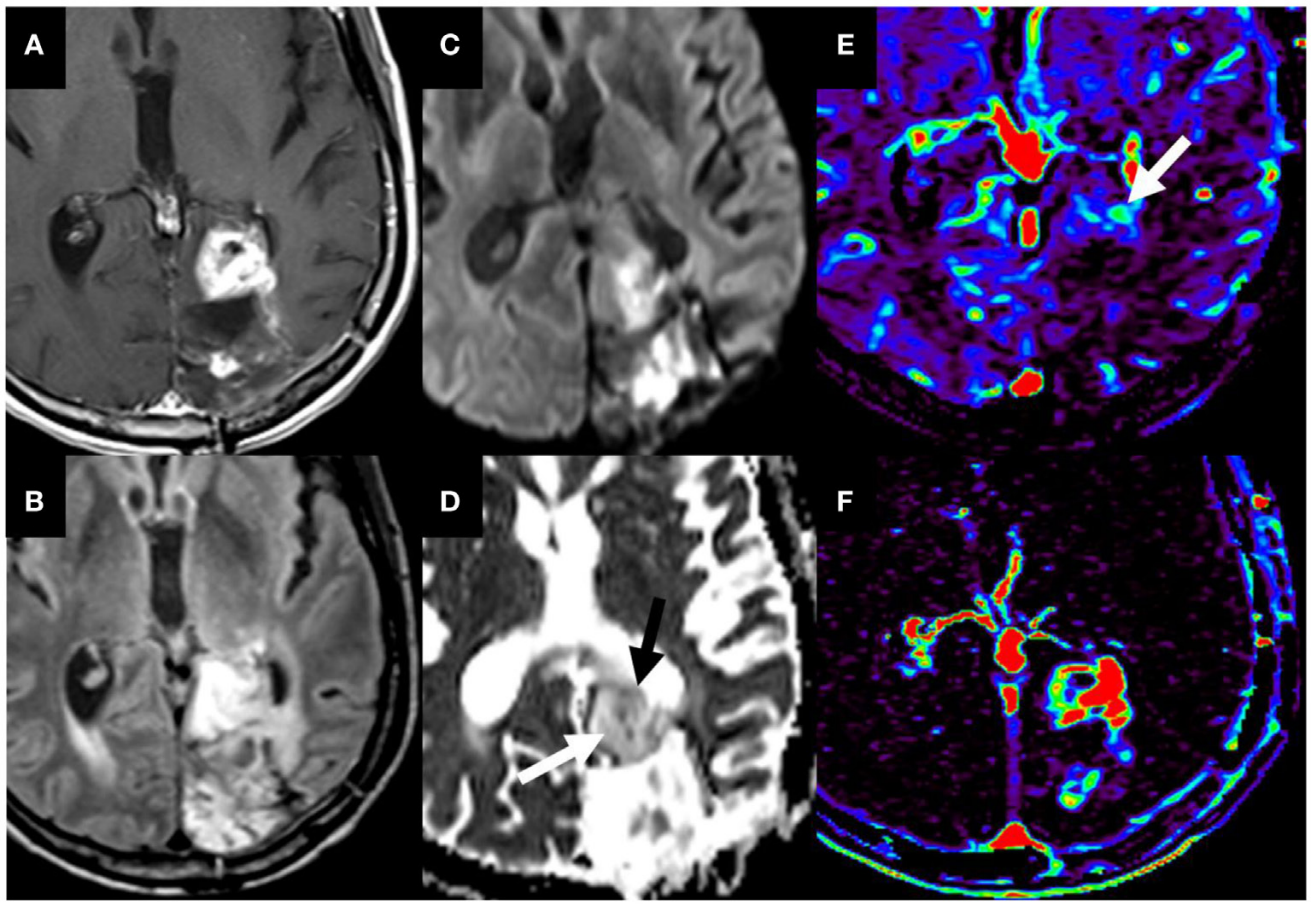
T1W postgadolinium (A) and T2W FLAIR (B) magnetic resonance (MR) sequences demonstrate a region of heterogeneous mass-like enhancement at the anterior border a glioblastoma resection cavity in the left occipital lobe that had progressed since the prior study. Diffusion-weighted imaging (C) and the corresponding ADC map (D) demonstrate predominately increased water motion suggesting necrosis [(D), white arrow]. The dynamic susceptibility contrast maps demonstrate predominately decreased microvascularity on the Vp map (E) with markedly increased permeability on the K-trans map (F). However, there is a smaller internal region of restricted diffusion [(D), black arrow] and increased Vp [(E), white arrow]. The combination of these imaging findings, diffusion and perfusion metrics are most compatible with pseudoprogression/treatment changes with a smaller internal region of recurrent tumor. These findings were confirmed on pathology. No copyright permissions were required to use these images.
